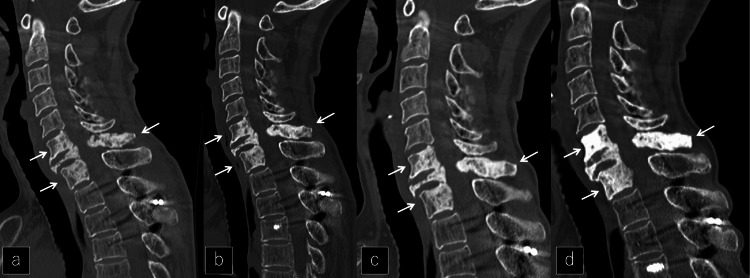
Changing image over time on CT for five years a, b, c, d: Sagittal CT at 3 months, 1 year, 2 years, and 5 years. Sclerotic change progressed in the affected vertebrae (white arrows).
